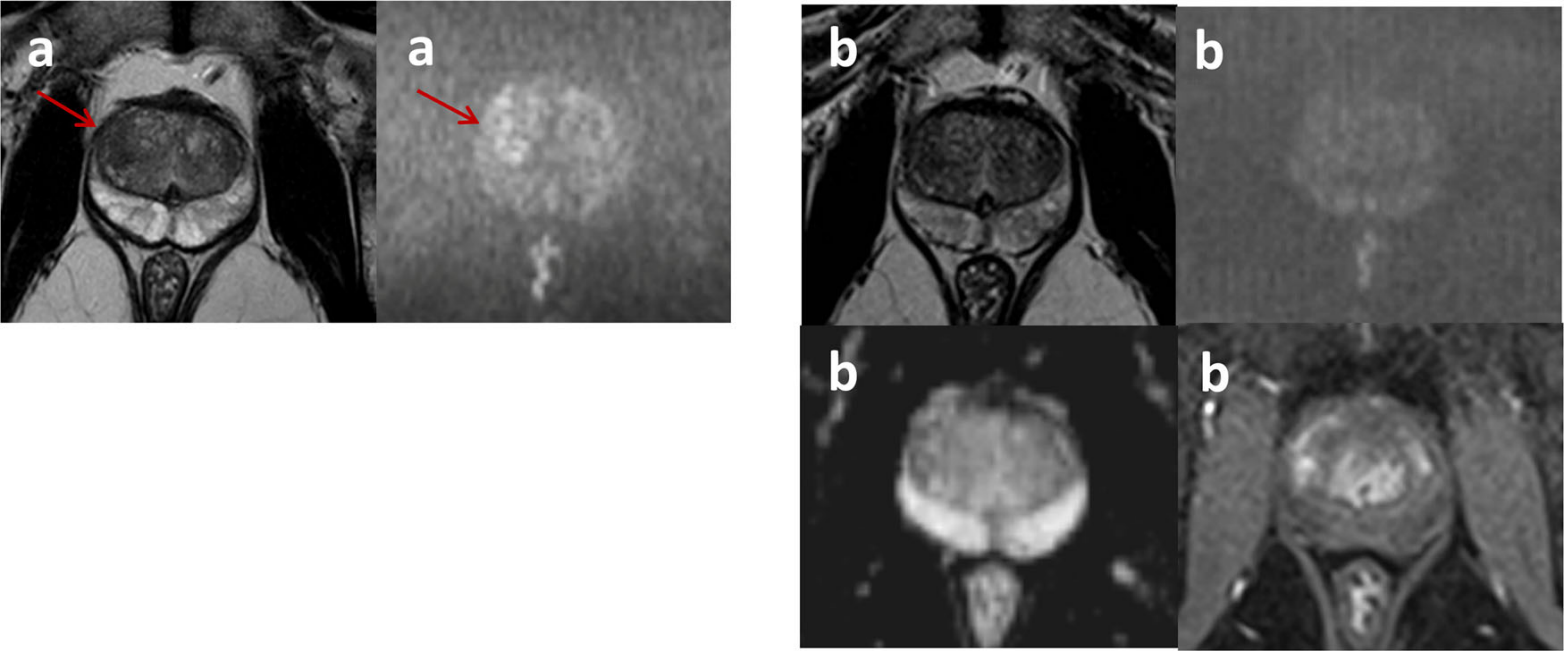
Example of a screening MRI-false-positive scan: (a) paired T2WI (left) and b2000 DWI (right) from the screening MRI of a 64-year-old male, which was reported as concordant positive due to a lesion in the right transition zone (arrow). There is a focal high signal on DWI but only a subtle change on T2WI. PSA was 0.81 ng/mL and PSAd 0.03 ng/mL^2^. b T2WI (top left), DWI (top right), ADC map (bottom left), and early dynamic contrast-enhanced images (bottom right) from the subsequent mpMRI show no focal abnormality. This was classified as a borderline overcall/limitation of the scoring system
